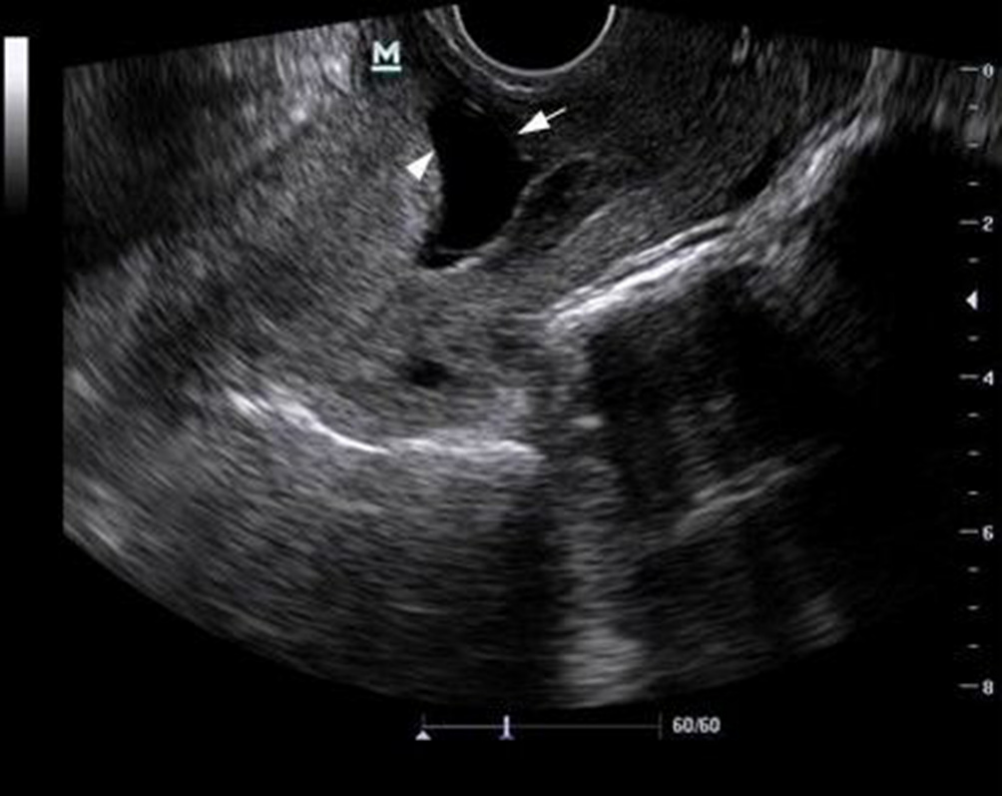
Transvaginal sonography showing an empty uterine cavity with a gestational sac located in the anterior myometrium of the lower uterine segment (solid arrow). The examination also demonstrates a thin anterior myometrium (arrowhead) with no fluid collection in the posterior cul-de-sac.
